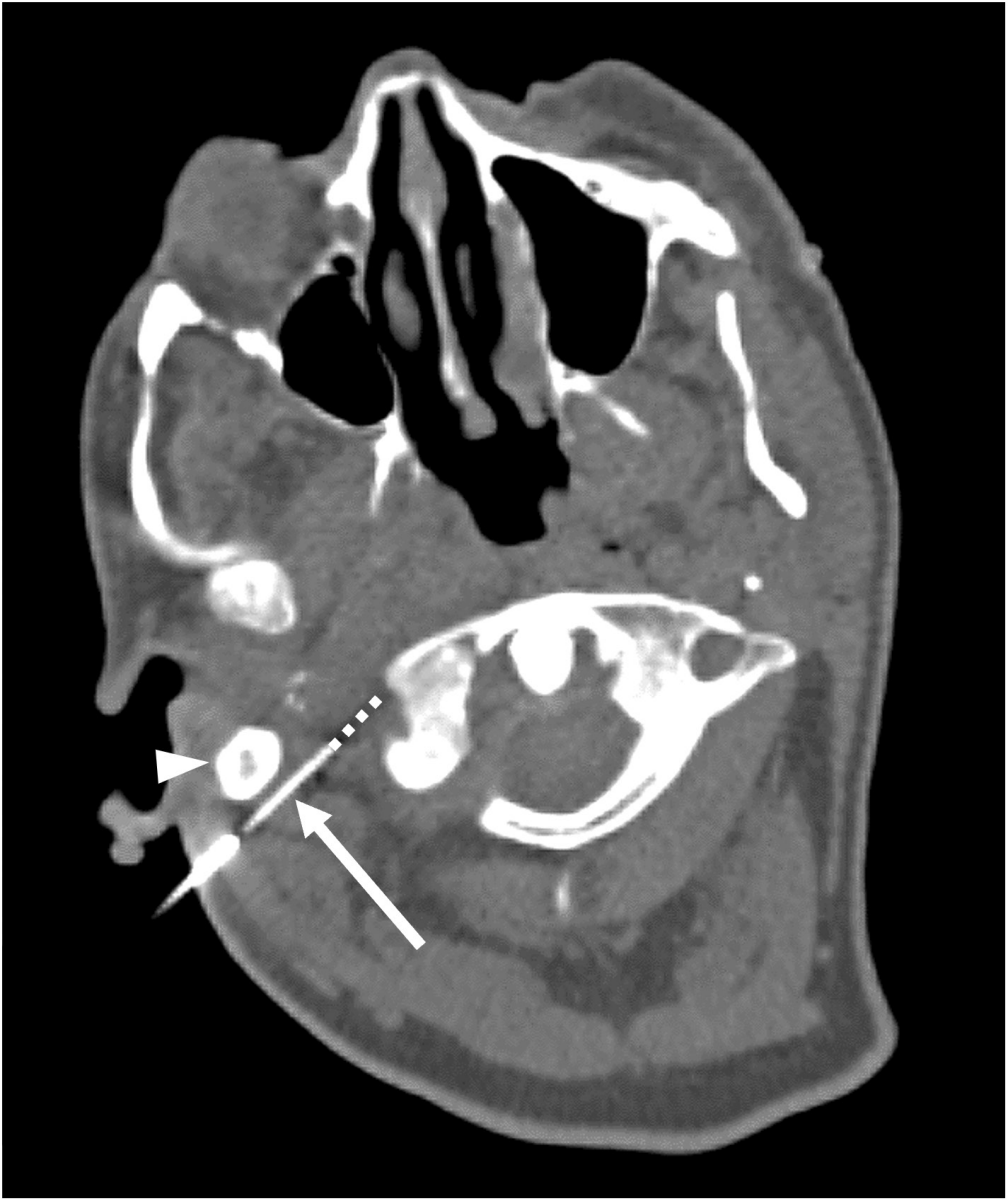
Percutaneous CT‐guided biopsy imaging demonstrating the retromastoid (wedge) course (dashed line) of core biopsy needle (arrow) to sample skull base lesion.
